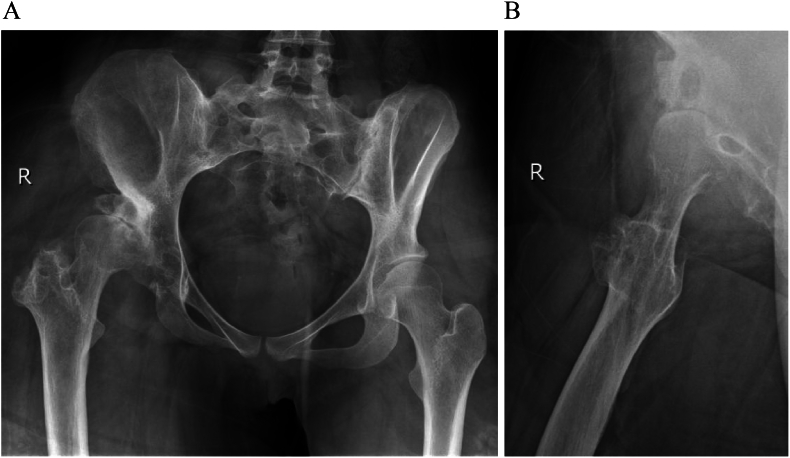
X-ray shows dysplasia and subluxation of the right hip (Crowe III).
